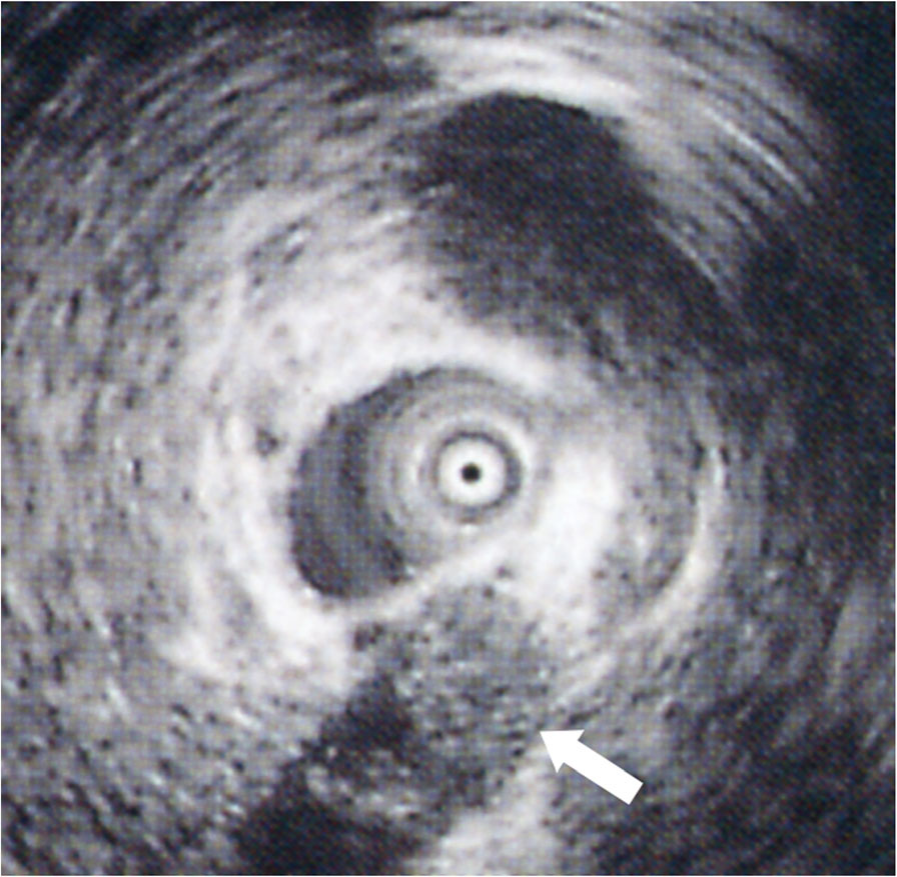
Endoscopic ultrasonography shows mass lesion in the remnant cystic duct. (From Nakamura A, Yagi K, Sekine A, Nihei K, Oyamatsu M, Tamiya Y. An early cystic duct carcinoma with hemobilia: Report of a Case. Journal of Gastroenterological Imaging [in Japanese] 2002;4(5):593–7; with permission)
